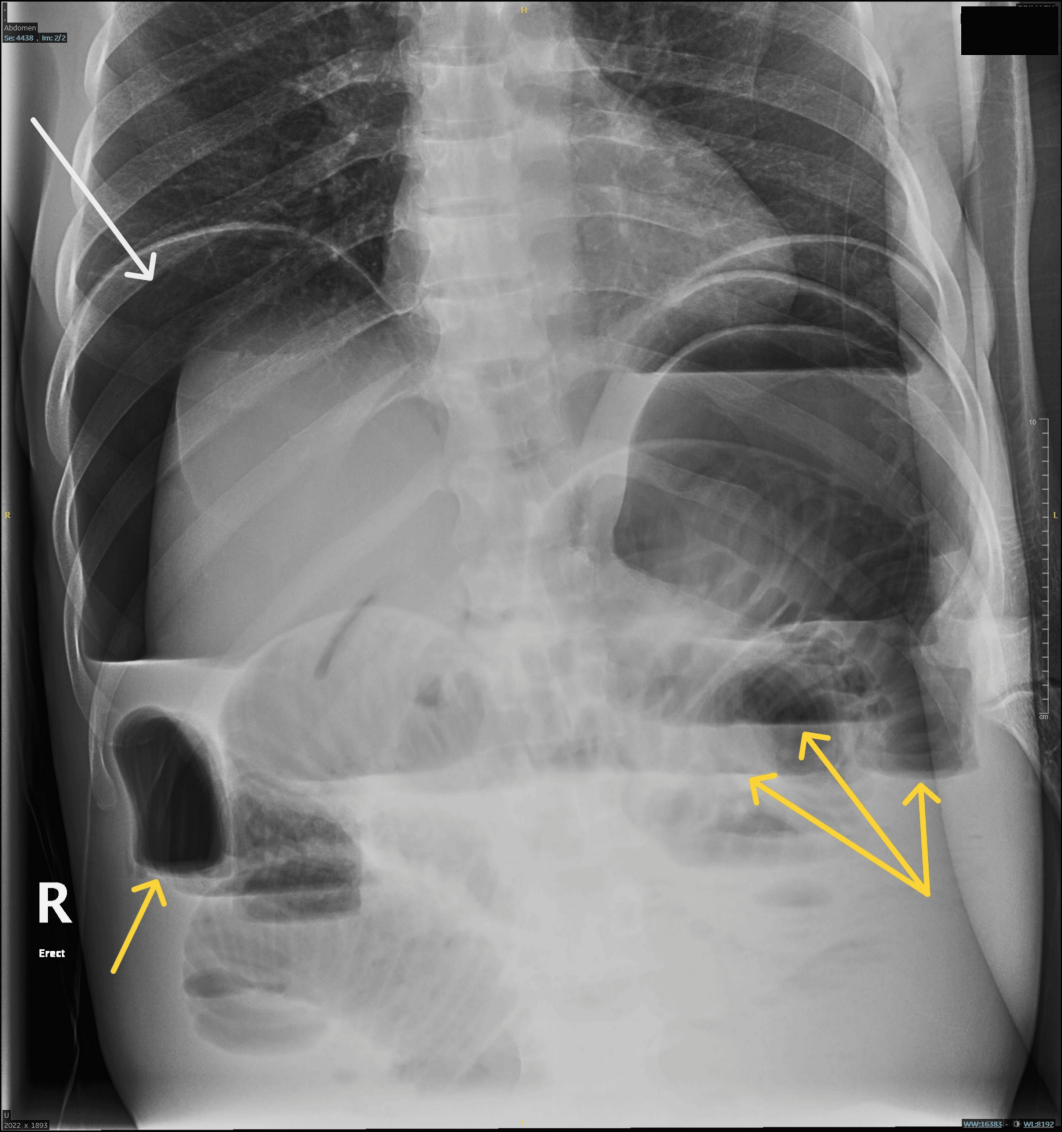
Plain X-ray abdomen The image is showing pneumoperitoneum (white arrow) and multiple air fluid levels (yellow arrows).
